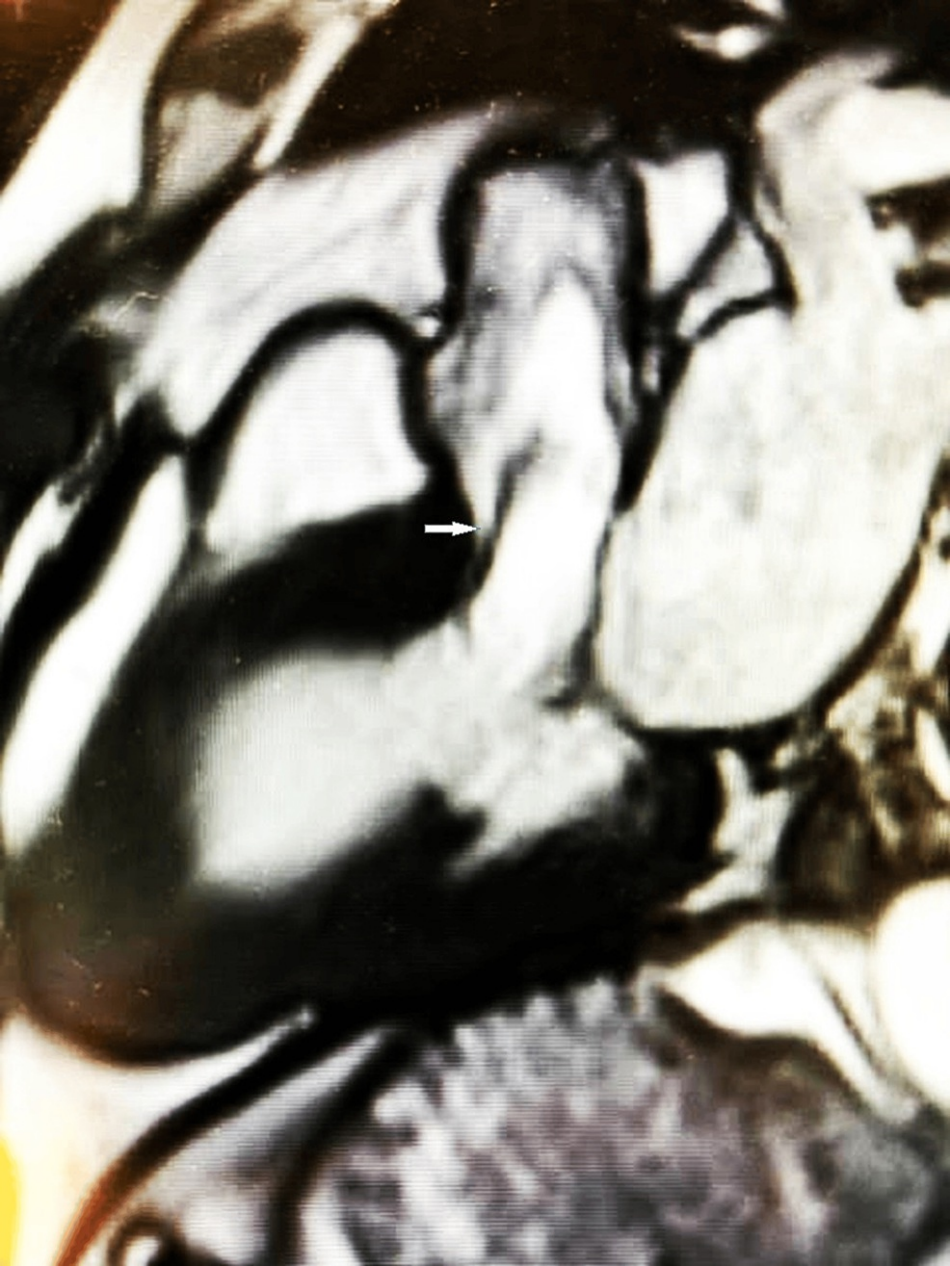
Cardiac MRI showing a sub-aortic membrane (white arrow)
